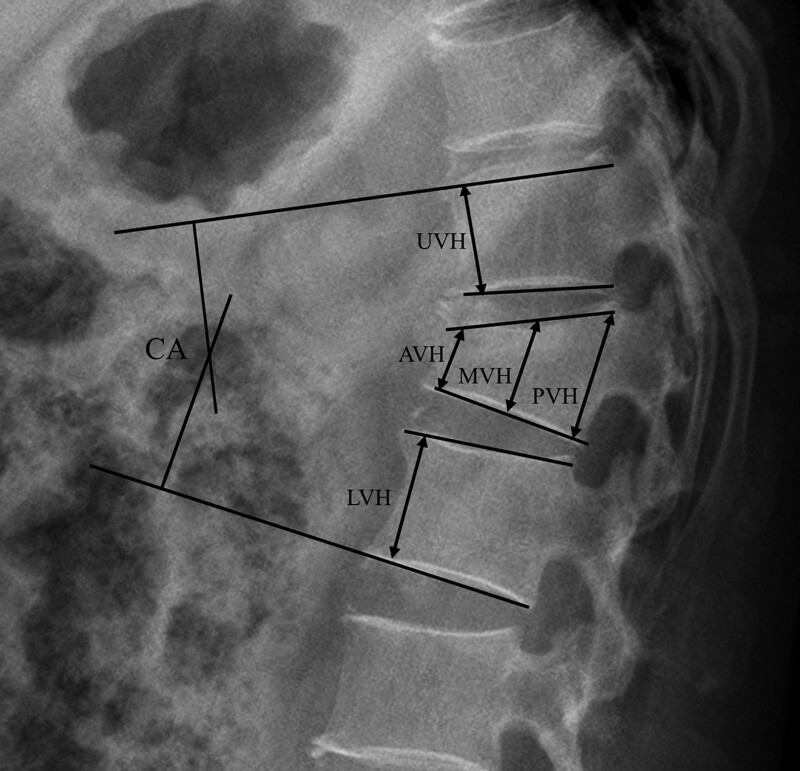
Parameters measured on a lateral radiograph. The vertebral compression ratio was calculated by the following formula: (1–[2 × AVH/UVH + LVH]) × 100. AVH = anterior vertebral height, CA = Cobb angle, LVH = lower vertebral height, MVH = middle vertebral height, PVH = posterior vertebral height, UVH = upper vertebral height.
